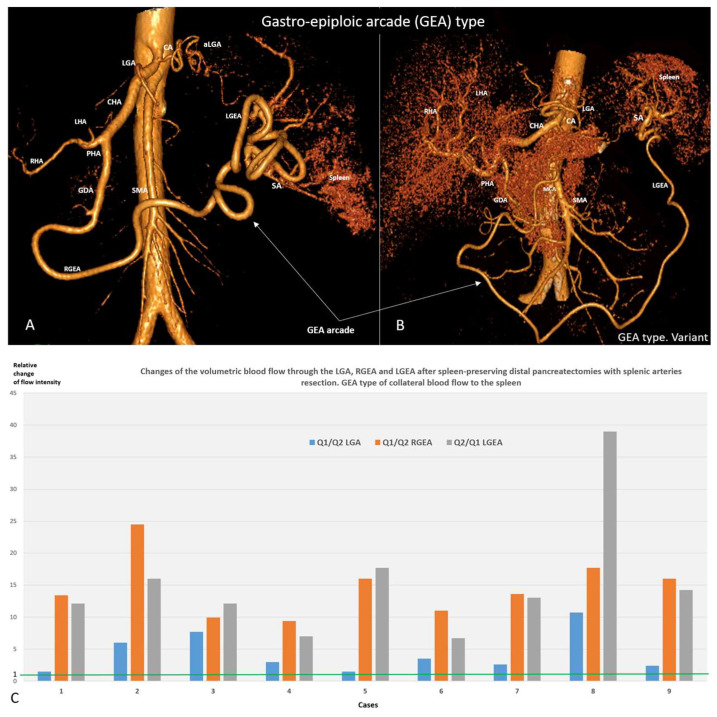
GEA type of collateral blood supply to the spleen after SPDP SVR. (A,B) CTA demonstration of the gastro-epiploic arcade (GEA) type of blood supply to the spleen after SPDP SVR for mucinous cystadenomas in 25- (A) and 41- (B) year-old women. The short gastric arteries, and through them, the spleen, receive blood from the gastroepiploic arcade. The gastroepiploic arcade, which was open before surgery, is now closed. In case A, the trunk of the SA is fully excised, and the diameter of the LGA after surgery increased insignificantly and is half the diameter of the RGEA. In both cases, RGEA and LGEA increased their diameters by two times, increasing the VBF through each artery by 16 times after surgery. In case B, six cm of the SA trunk was excised and RGEA originated from the middle colic artery, which is a described but rare variant. (C) The chart shows the calculated relative change in blood flow intensity (volumetric flow rate in mL/min, Q) through the LGA, RGEA and LGEA after SP DP. As shown, the blood flow intensity through the GEA can increase by 8–24 times and LGEA by 7–38 times (with regard to the baseline, i.e., the 1.0 value), which underlines the adaptive ability of the GE arcade and its hemodynamic contribution to this type of collateral blood supply to the spleen. The CTA shows cases #2 and #5.
